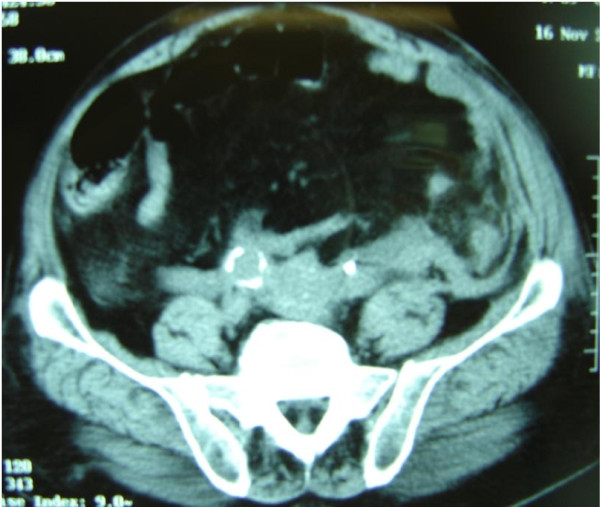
Preoperative CT scan showing diffuse retroperitoneal fibrosis.
